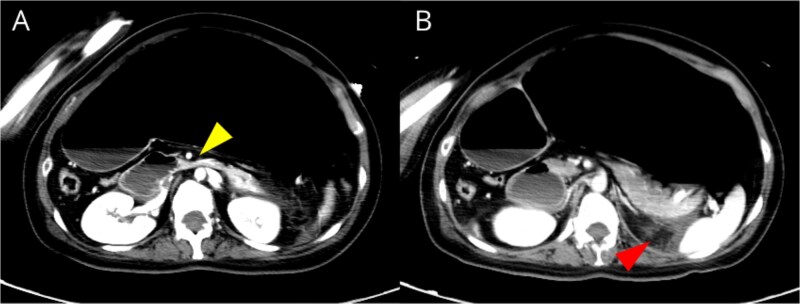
(A) Axial contrast-enhanced computed tomography image showing compression of the third portion of the duodenum between the aorta and the superior mesenteric artery, with marked proximal dilatation of the stomach and duodenum. (B) Contrast-enhanced computed tomography image showing peripancreatic fat stranding extending to the inferior pole of the left kidney, without evidence of pancreatic necrosis.
